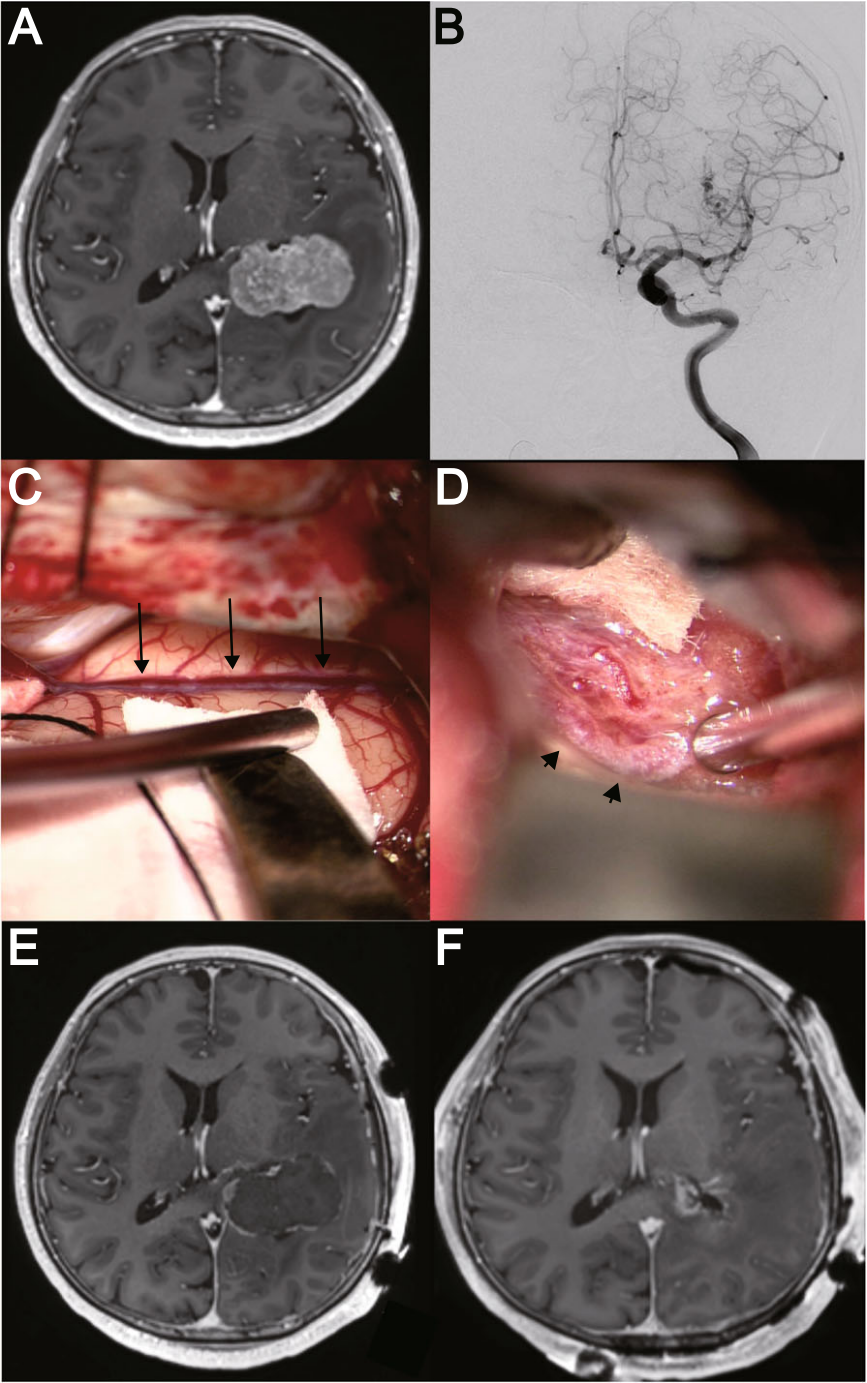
In Patient 3, a left lateral ventricular tumor was incidentally detected eight years prior. Since the patient was experiencing visual field impairment due to tumor growth, surgical intervention was planned. a Preoperative magnetic resonance imaging (MRI) scan with gadolinium contrast showing a tumor in the left lateral ventricle (maximum diameter: 5.1 cm, tumor volume: 27.8 cm^3^). b Preoperative digital subtraction angiography (antero-posterior projection) reveals feeders originating solely from the anterior choroidal artery. The diameter of the AChA was 0.9 mm, which was considered too small to cannulate. c After temporal craniotomy, the occipitotemporal sulcus (black arrows) has been identified. The temporal horn is accessed by a subtemporal approach. d In the temporal horn, the anterior choroidal artery has been identified (black arrowheads), and cut. e After microsurgical occlusion of the anterior choroidal artery, MRI shows a 98.6% decrease of gadolinium-enhanced lesions. f After tumor removal via a parietal (intraparietal sulcus) approach, MRI shows no residual tumor
